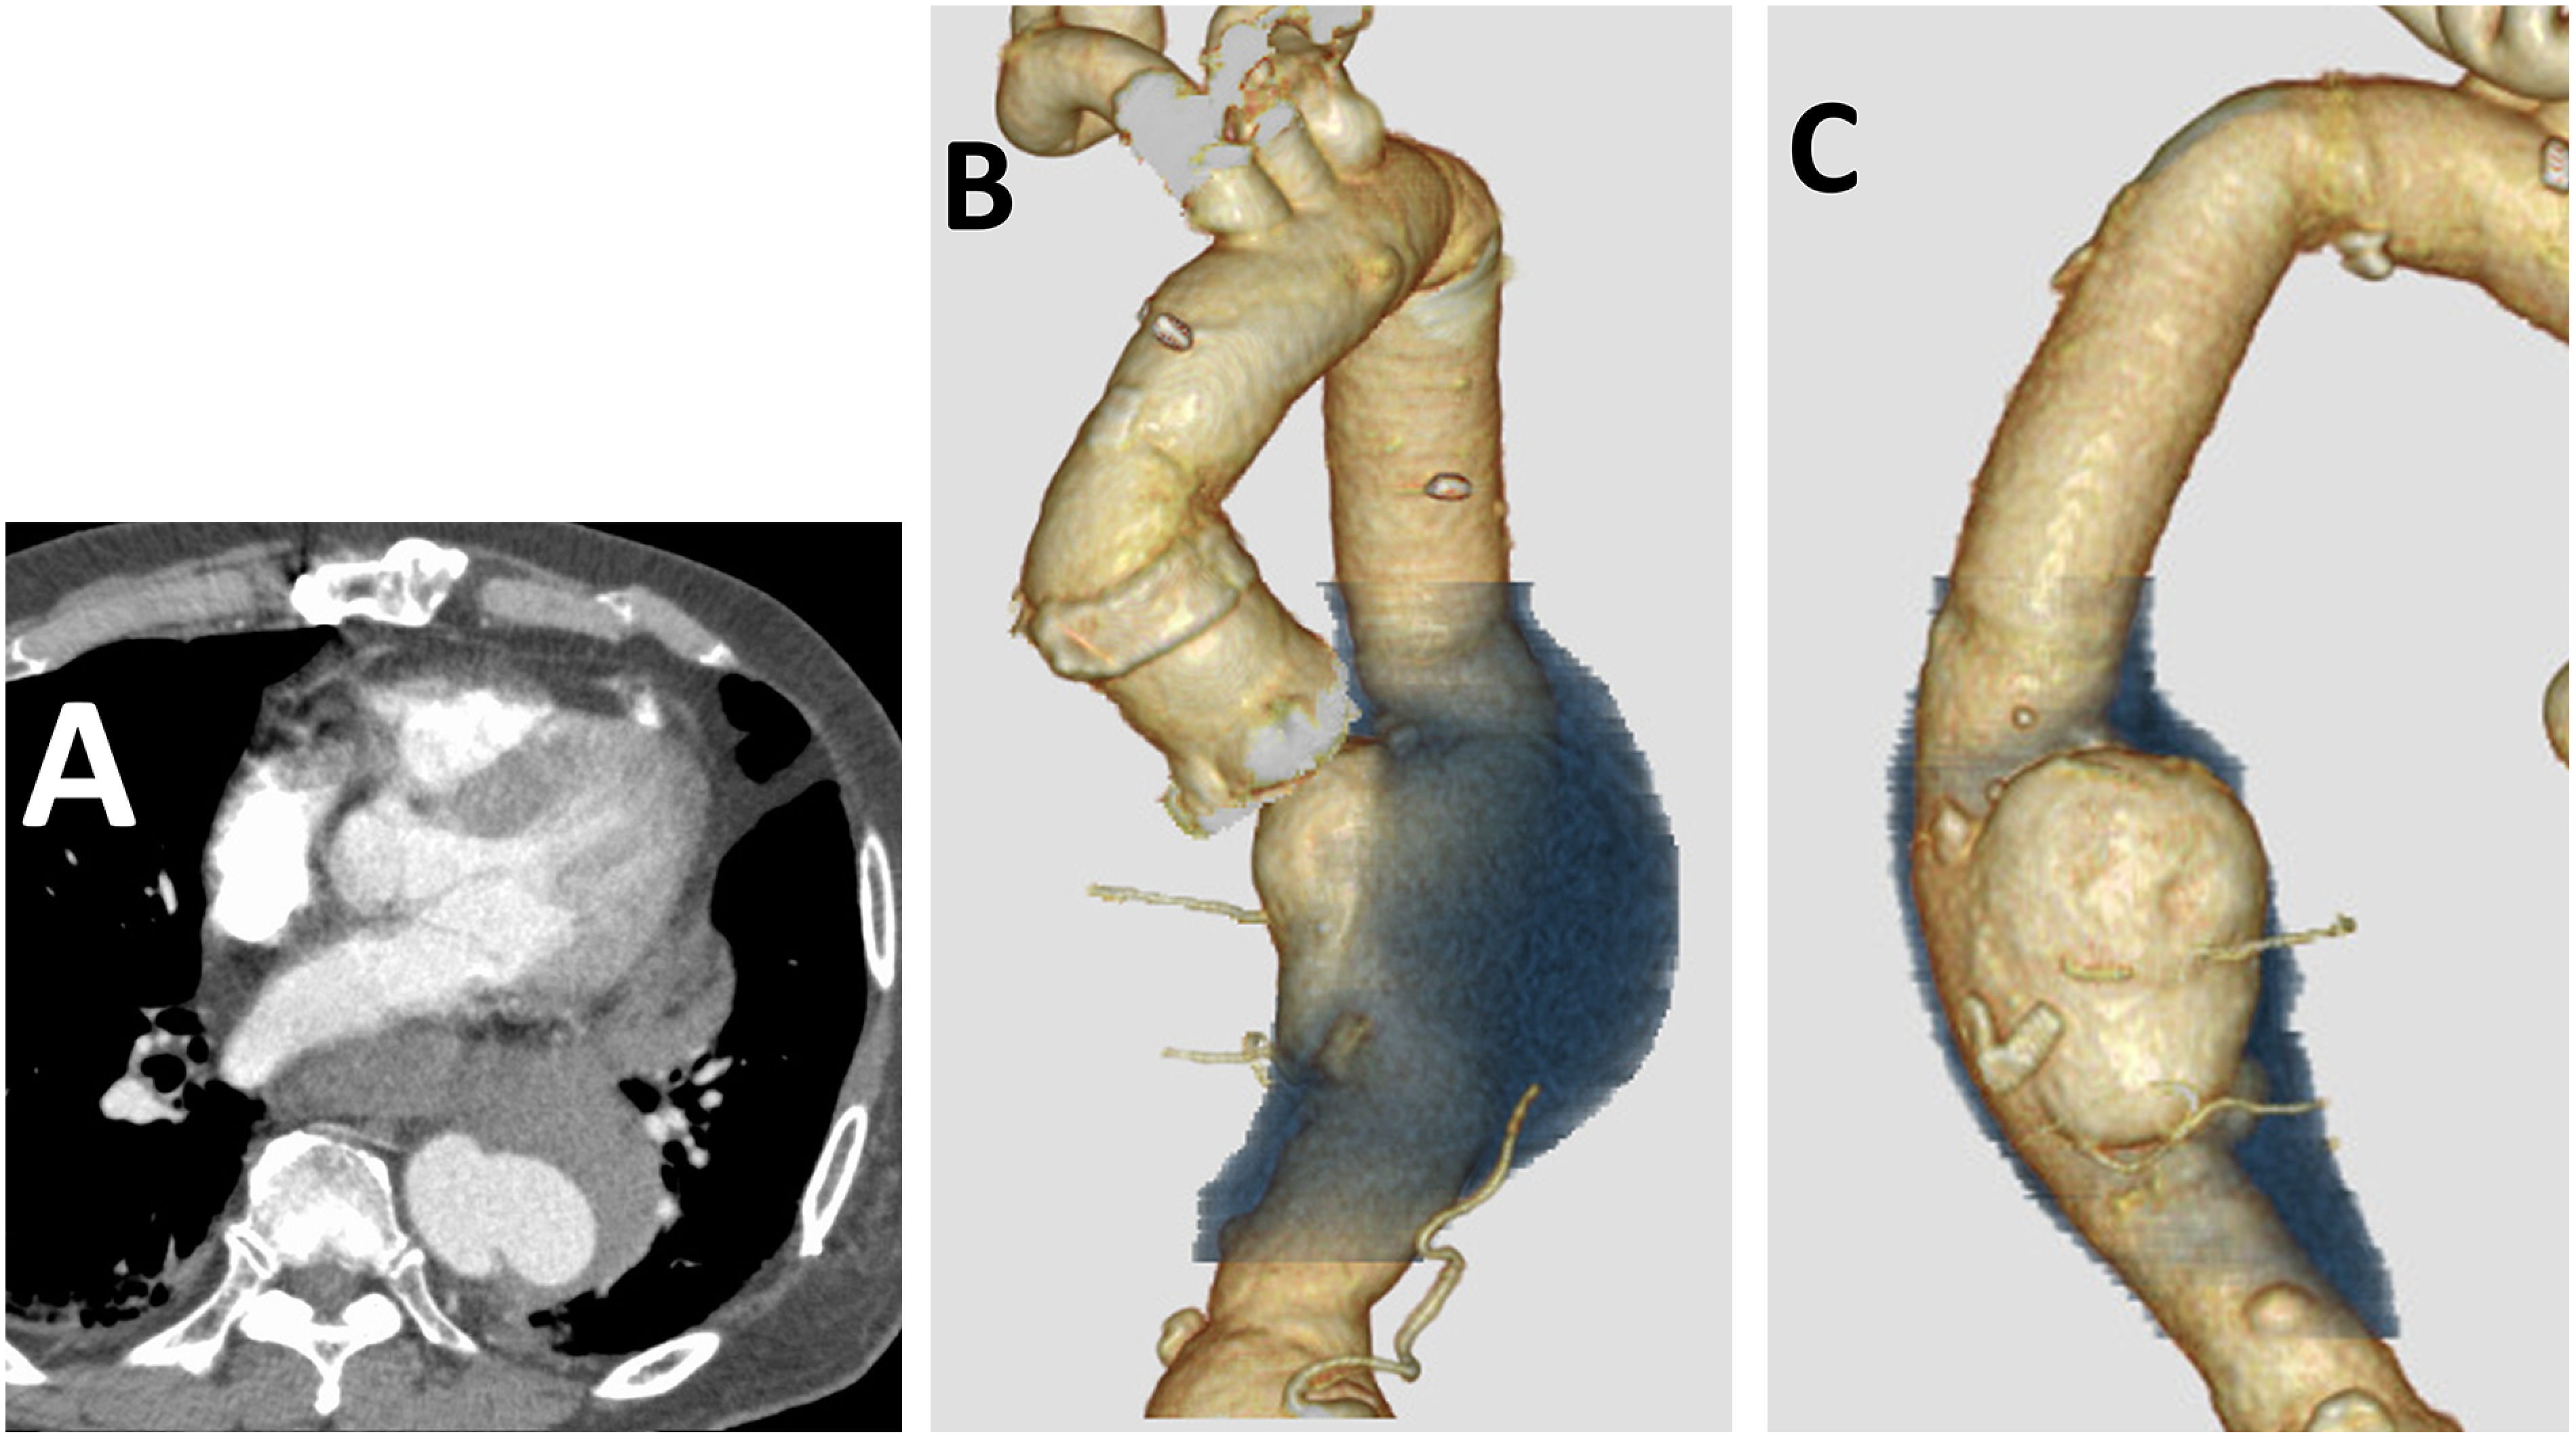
Fig. 1 Preoperative enhanced computed tomography scan showing an intercostal patch aneurysm measuring 64×60×61 mm with rupture and a huge hematoma. (A): Axial view; (B), (C): 3-dimensional view.
